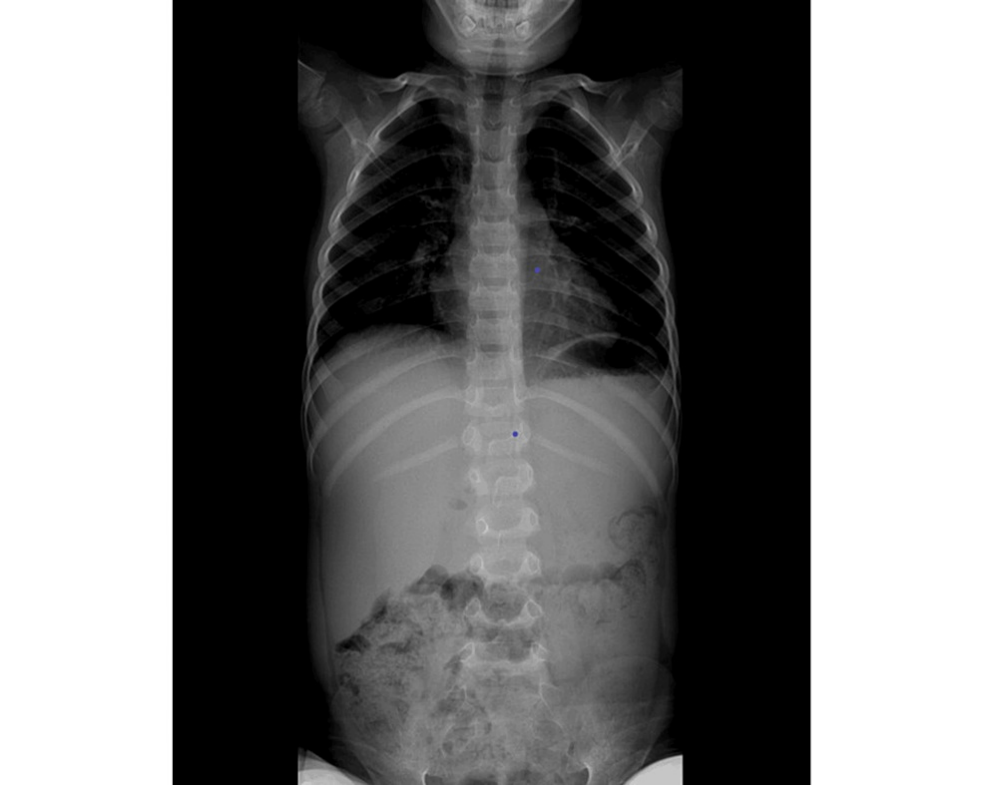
Coronal view of the long spine X-ray. A slight dorsolumbar scoliotic attitude with left convexity centered at L2-L3, although difficult to assess, was not associated with evident body rotation.
